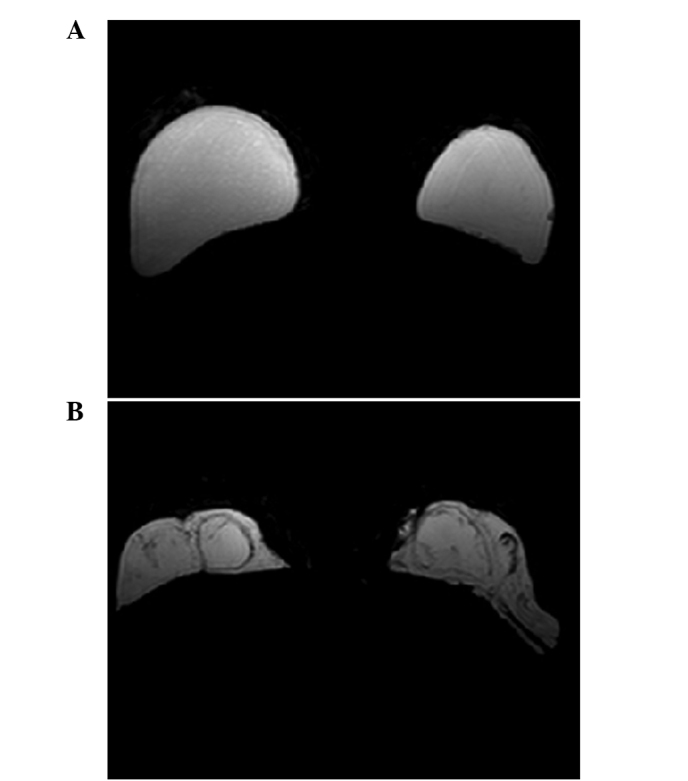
Axial T2-weighted MRI scans with fat depression of PAHG infiltrated in various layers. (A) Patient 8. PAHG was injected into the subglandular space and distributed regularly. (B) Patient 1. PAHG infiltrated into the surrounding tissue and was distributed irregularly. MRI, magnetic resonance imaging; PAHG, polyacrylamide hydrogel.
